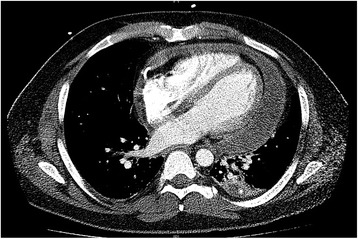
Axial computed tomography scan of chest showing pericardial effusion
